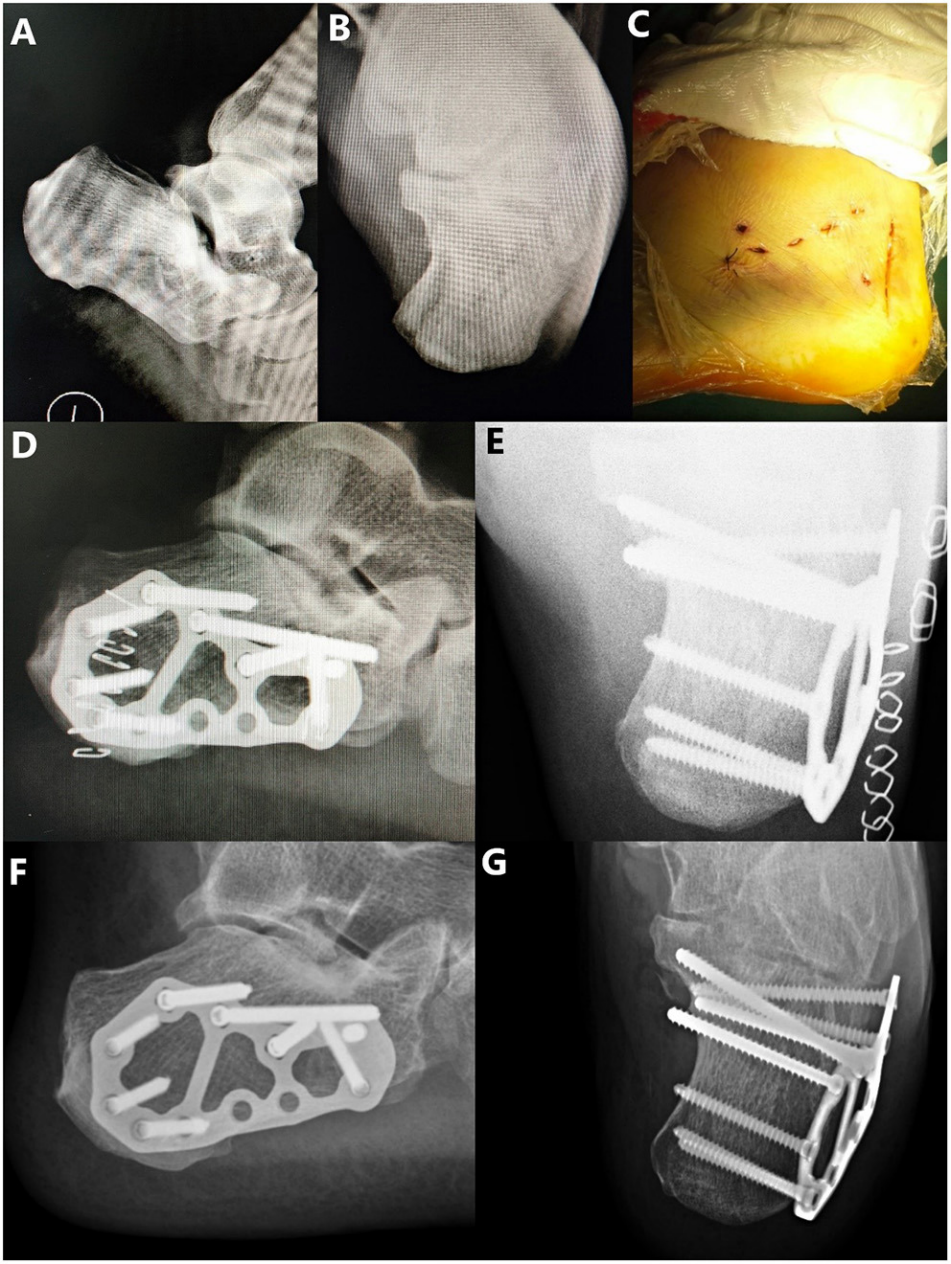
A 31-year-old male patient who fell from a height, resulting in a tongue-type intra-articular fracture of the calcaneum. (A) Preoperative lateral X-ray and (B) preoperative axial X-ray presenting an intra-articular tongue-type calcaneal fracture with subtalar articular surface collapse, loss of Böhler's angle and Gissane's angle, significantly decreased calcaneal height/length, and significantly increased calcaneal width. (C) Postoperative incision. (D) Postoperative lateral X-ray showing the reduction of the calcaneal length and height, Böhler's angle, and Gissane's angle. (E) Postoperative axial X-ray showing the reduction of the calcaneal width. (F) Lateral X-ray 12 months after the surgery. (G) Axial X-ray 12 months after the surgery.
